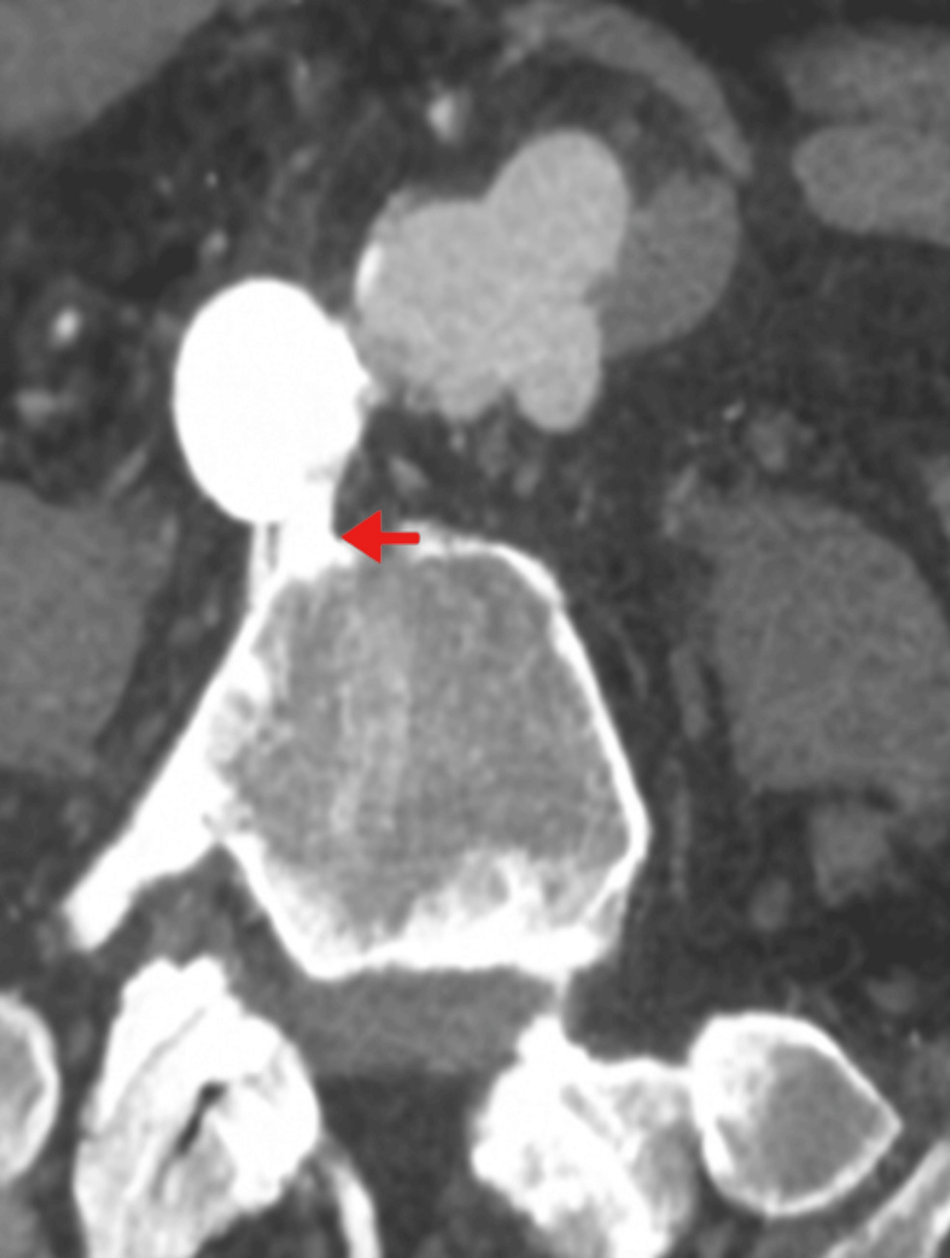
Abdominal CT angiography showing an aortic distal anastomotic pseudo-aneurysm with an aortoenteric fistula (red arrow).
